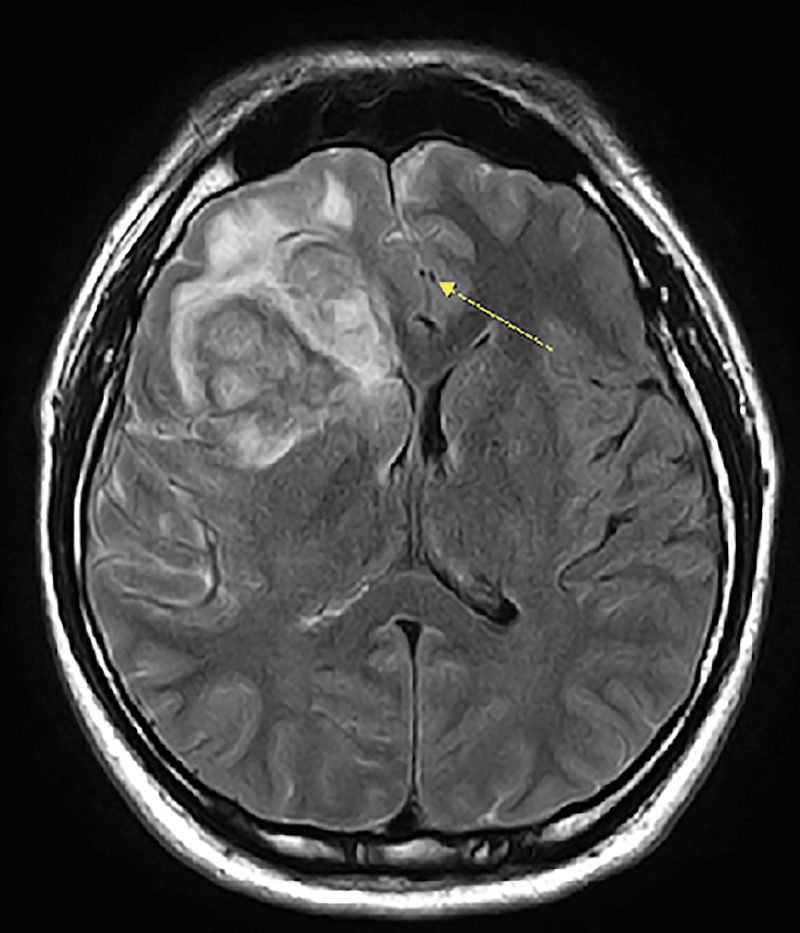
MRI T2 FLAIR showing the tumor location. The PTV definitely includes the bilateral anterior communicating artery (yellow arrow). MRI = magnetic resonance imaging.
